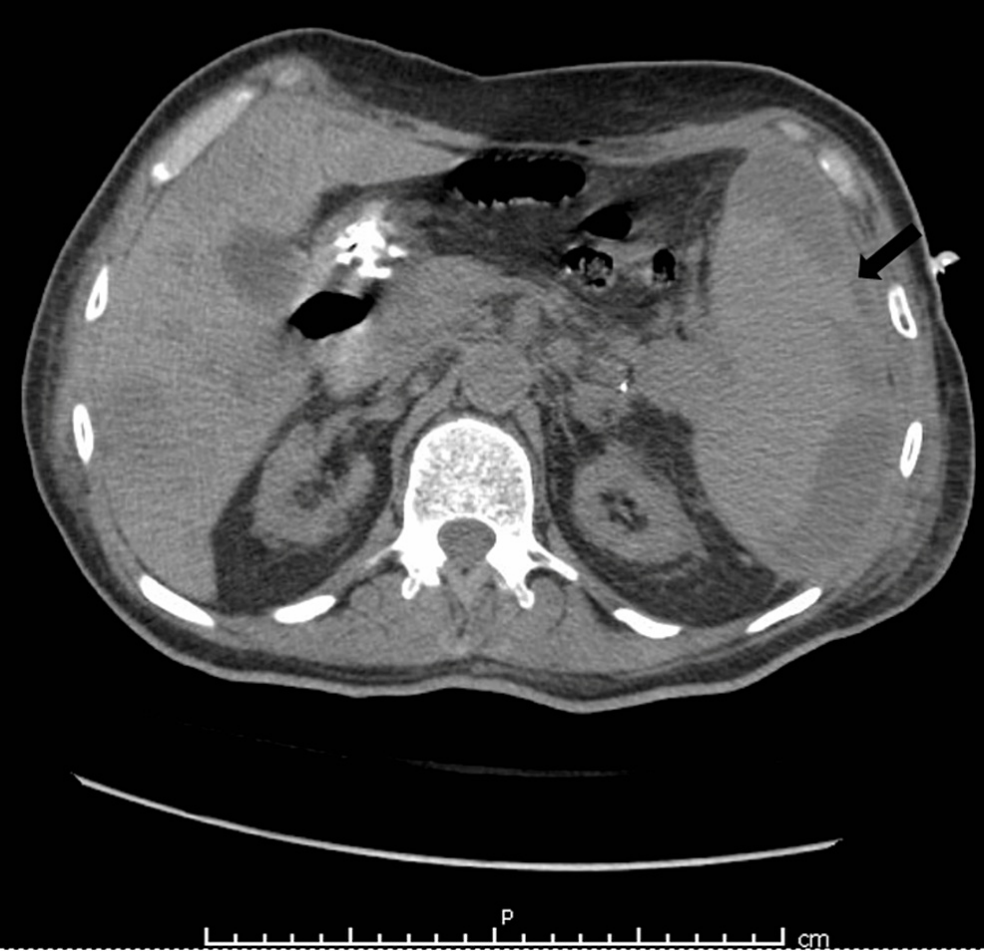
Computed tomography scan of the abdomen showing an enlarged spleen (arrow) with areas of hypoattenuation.
